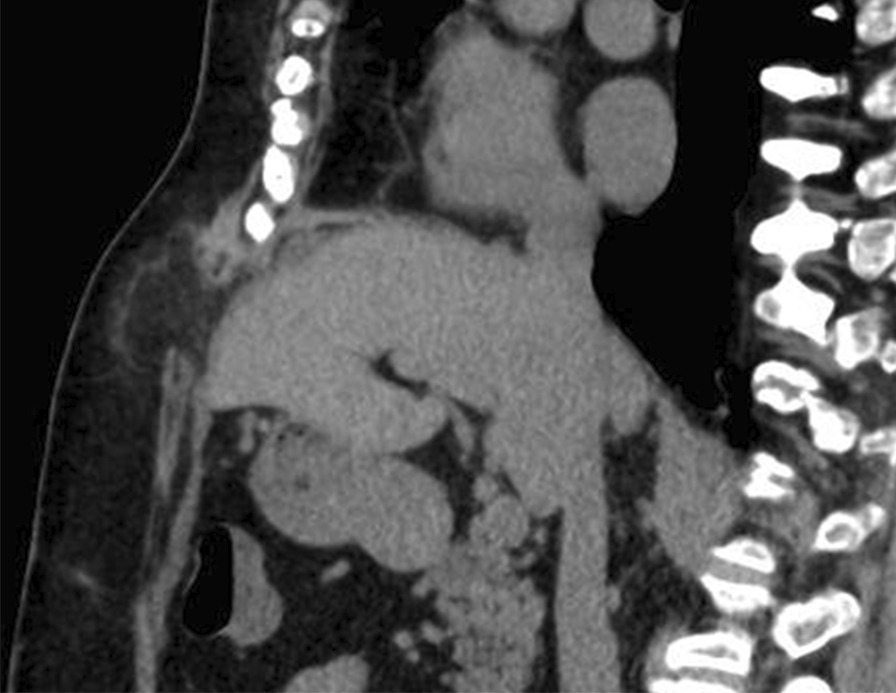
Abdominal computed tomography after 6 months. No recurrence has been observed
